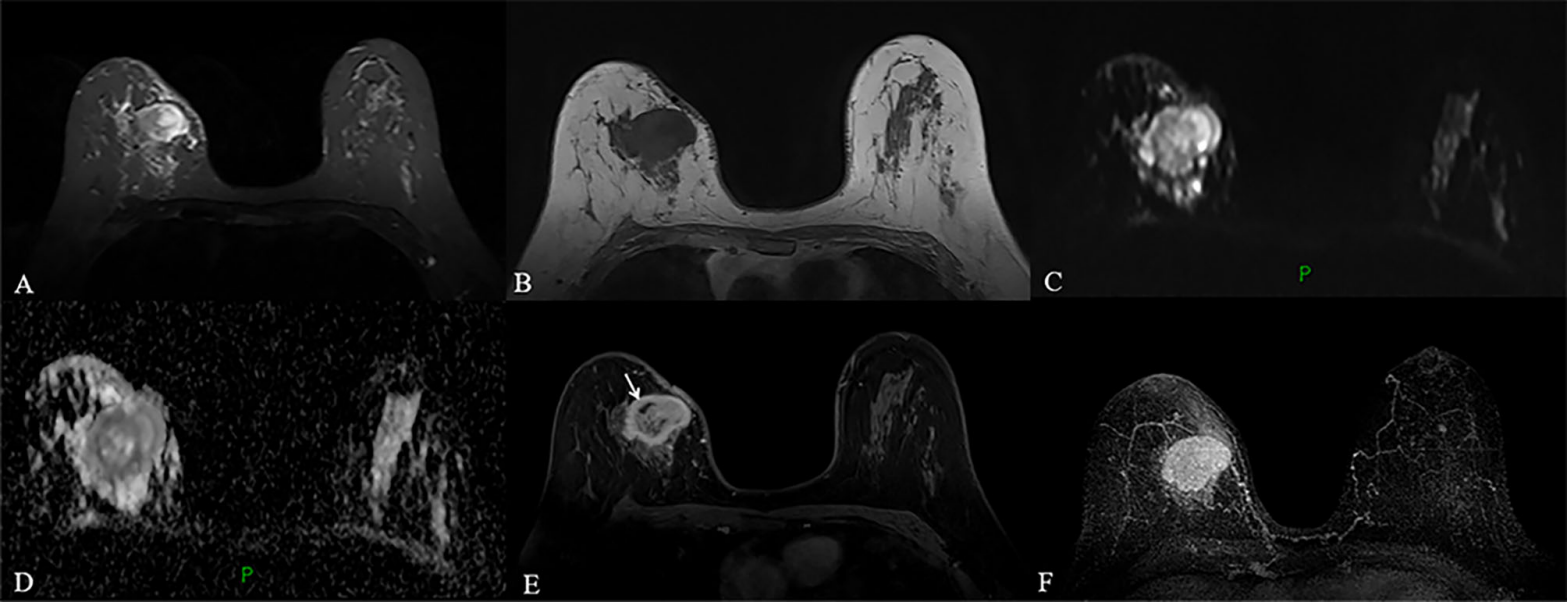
(A–F) MRI obtained two years after the operation revealed a circumscribed mass with irregular shape and slightly distorted structure, accompanied by thickening of adjacent skin in the inner upper quadrant of the right breast, a mass showed heterogeneous high signal intensity on fat-suppressed T2-weighted images and low signal intensity on T1-weighted images (A, B). The lesion with high signal intensity on DWI (C) had low signal intensity on ADC map (D), which indicated restricted diffusion. Dynamic contrast-enhanced MRI revealed a fast, heterogeneous enhanced mass in the initial phase (E). Maximum intensity projection (MIP) revealed a mass with increased blood supply (F).
